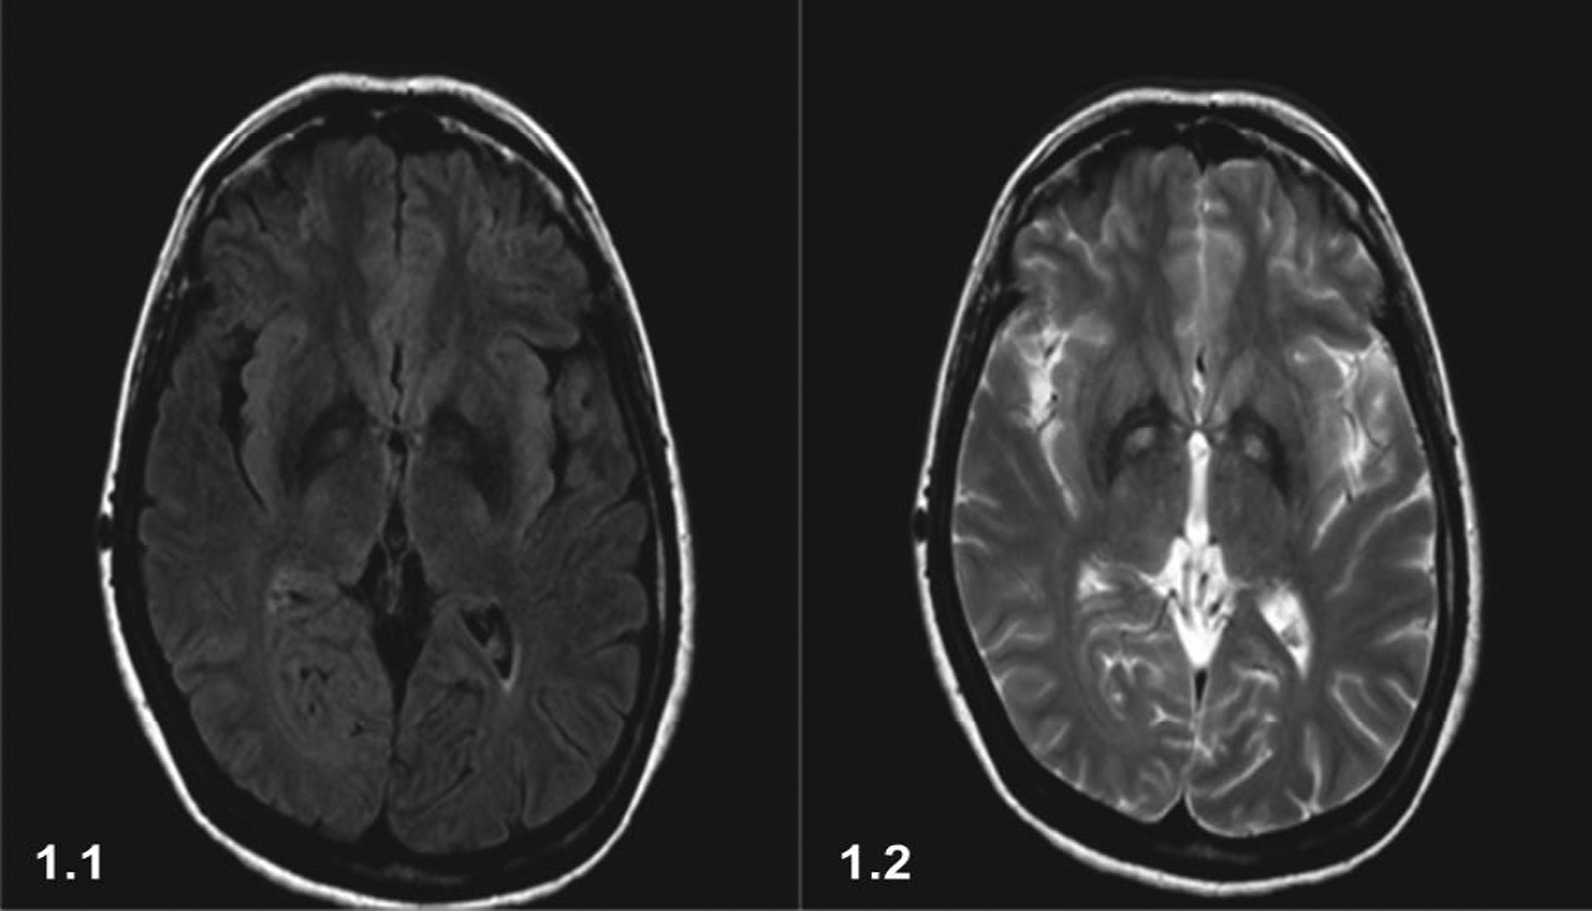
MRI brain showing T2 axial (1.1) and FLAIR (1.2) images: bilateral symmetrical hypointensity in globus pallidus with central hyperintensity “eye of the tiger” sign, due to pantothenate deficit
